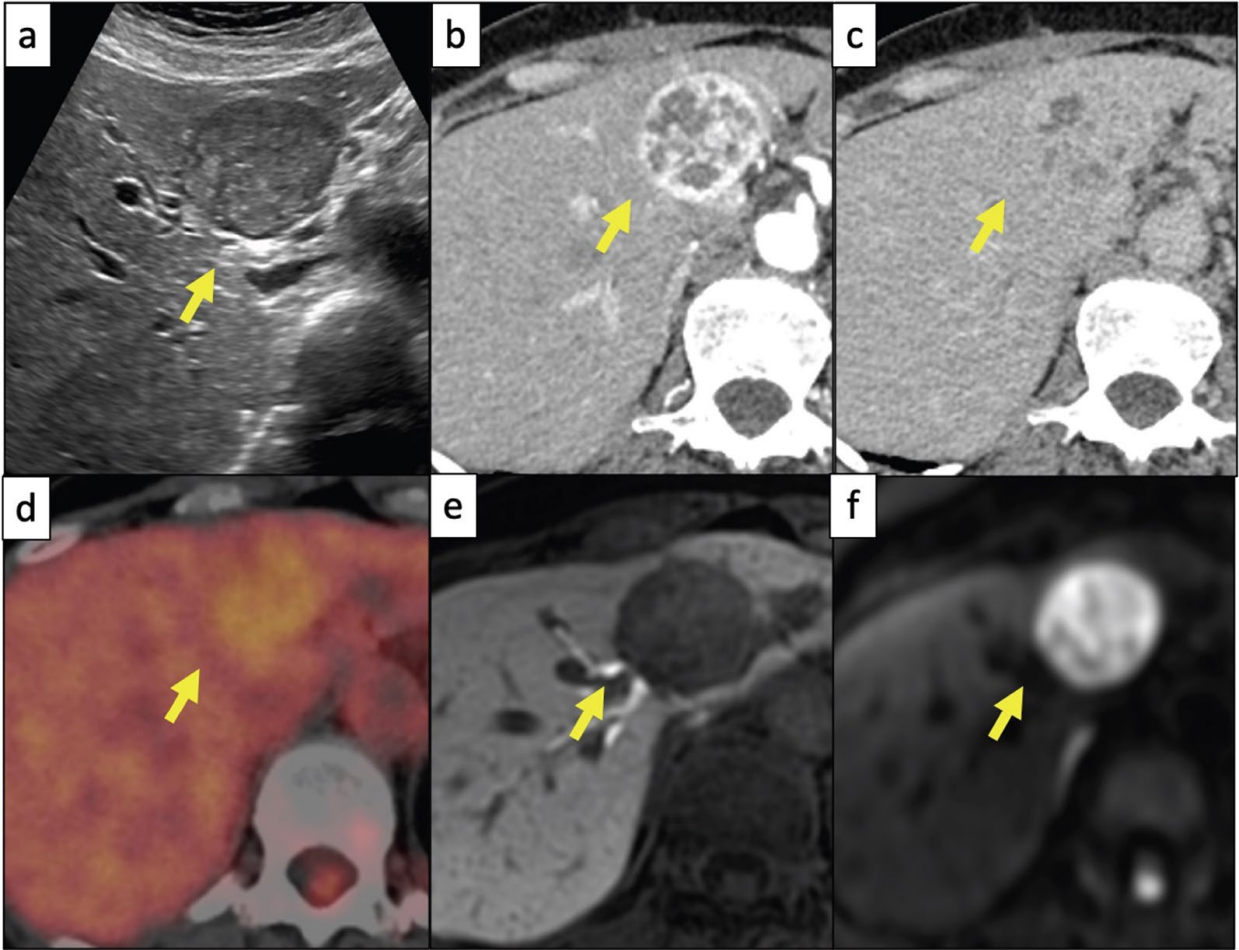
Preoperative imaging of a tumor. a Abdominal ultrasound showing a 4-cm mass in liver segment IV. b Tumor is heterogeneously enhanced with peripheral enhancement in the arterial phase of dynamic CT. c Early enhancing area is iso-intense to the liver parenchyma in the equivalent phase of dynamic CT. d FDG–PET shows slight hyperaccumulation in the tumor. e Signal of the tumor is markedly lower than that of the liver parenchyma in the hepatobiliary phase of gadolinium–ethoxybenzyl-diethylenetriamine penta acetic acid-enhanced MRI. f High signal intensity on diffusion-weighted image (*b* value of 1000 s/mm^2^) is noted. CT: computed tomography; FDG–PET: fluorodeoxyglucose–positron emission tomography; MRI: magnetic resonance imaging
